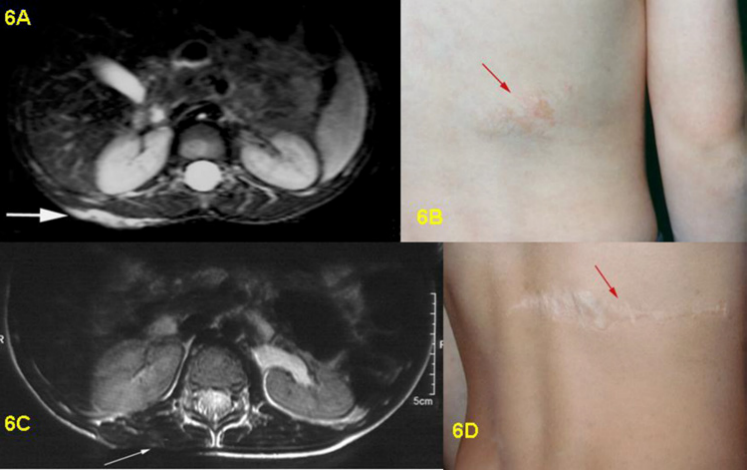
6A: Pre-surgical T2-weighted STIR-sequence, transversal section: bright, flat cutaneous and subcutaneous PNF of right back without involvement of abdominal wall and muscles. 6B: Pre-surgical clinical view of PNF on right back with hypertrichosis. 6C: Post-surgical T2-weighted Turbo Spin Echo-sequence, axial section: complete removal of PNF, thin scar, no subcutaneous fatty tissue visible in scan. 6D: Post-surgical clinical view of scar after tumor removal on back.
